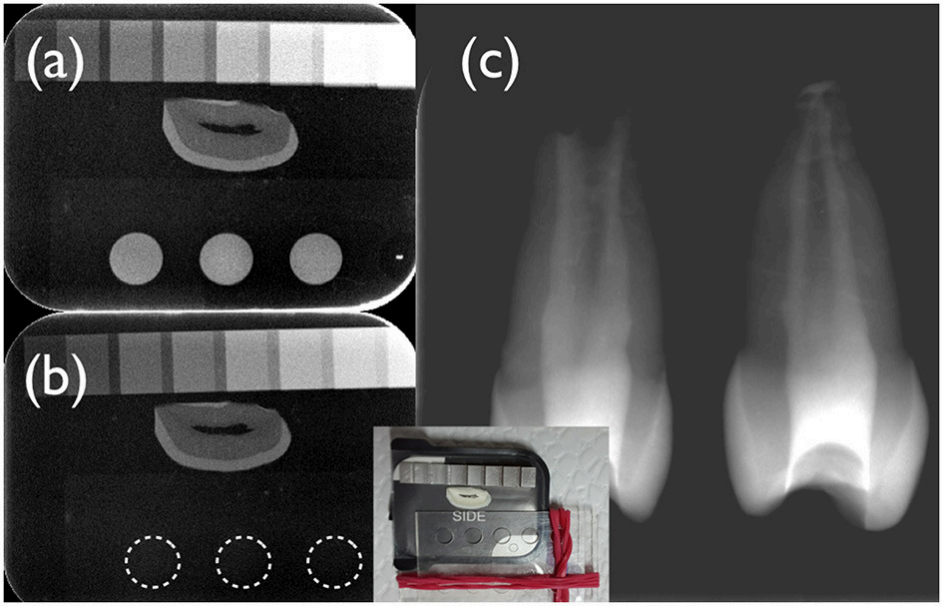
Digital radiographs of the test (a,c) and the control fibrin hydrogel (b) under investigation. A cross-section of a human incisor crown was used as a reference. A photograph of the set-up is depicted in the insert. The aluminum step-wedge had a thickness of 0.5, 1, 1.5, 2, and then 3 mm Al, followed by 1 mm steps. As can be appreciated from this figure, the test hydrogel (a) had a radiopacity of ~2 mm Al/mm, whilst the control hydrogel (b) displayed no radiopacity (dotted lines). Freshly mixed test hydrogel containing iodixanol was placed in the root canal system of immature human premolars using a lentulo spiral (c). This test hydrogel apparently filled the whole canal space and could be clearly discerned from root dentin.
